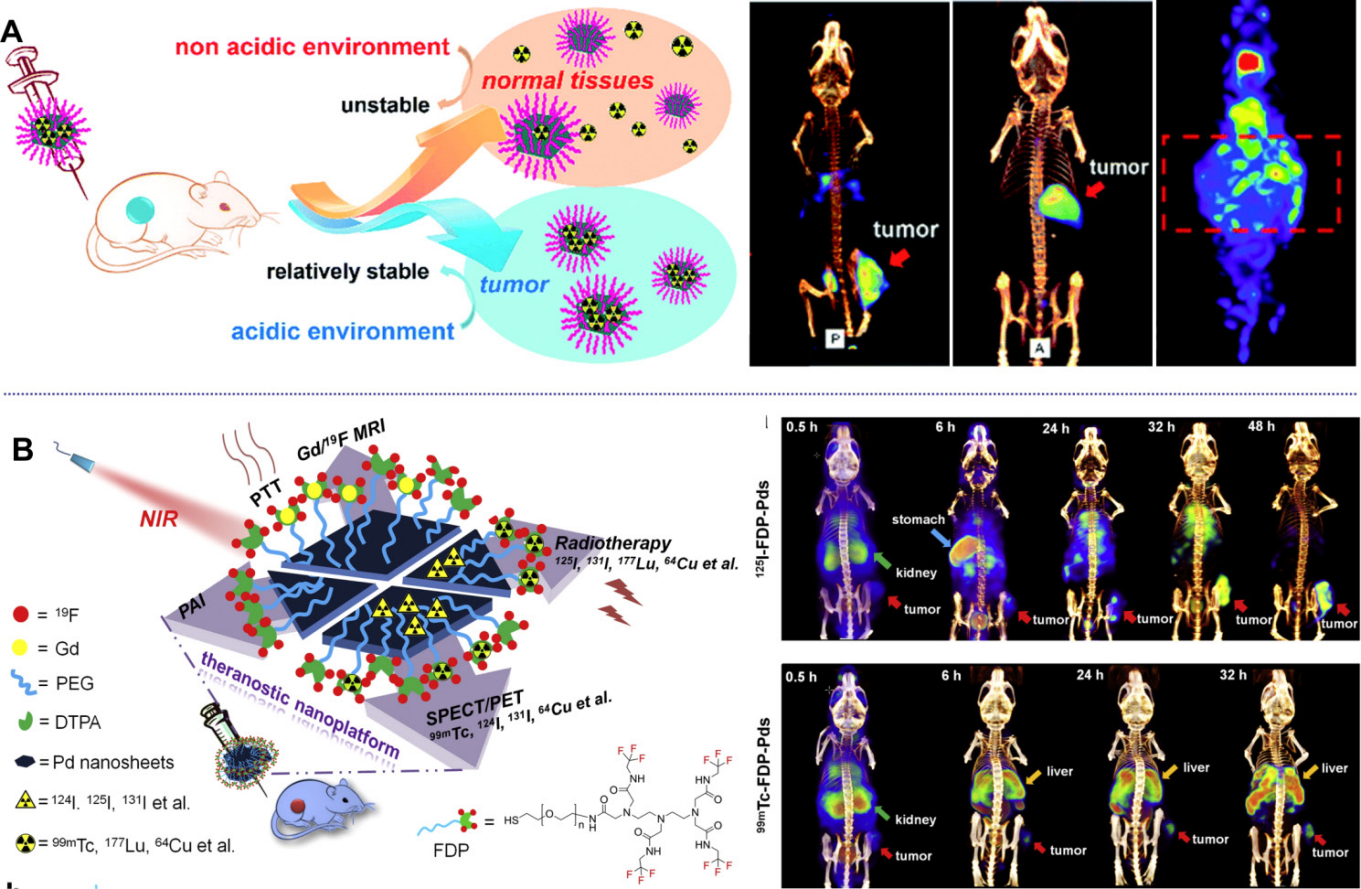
pH-sensitive radiolabeled Pd NSs for SPECT imaging in different tumor models, (A) radioactive iodide-labeled Pd NSs and (B) radioactive iodide and ^99m^Tc-labeled Pd NSs as a multifunctional theranostic platform. Adapted with permission from ref. 47. Copyright 2018, Royal Society of Chemistry; adapted with permission from ref. 48. Copyright 2018, Elsevier.
